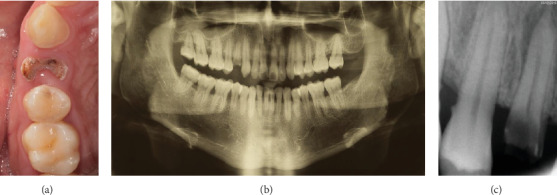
(a) Preoperative occlusal view; (b) orthopantomography; (c) preoperative periapical radiograph.
